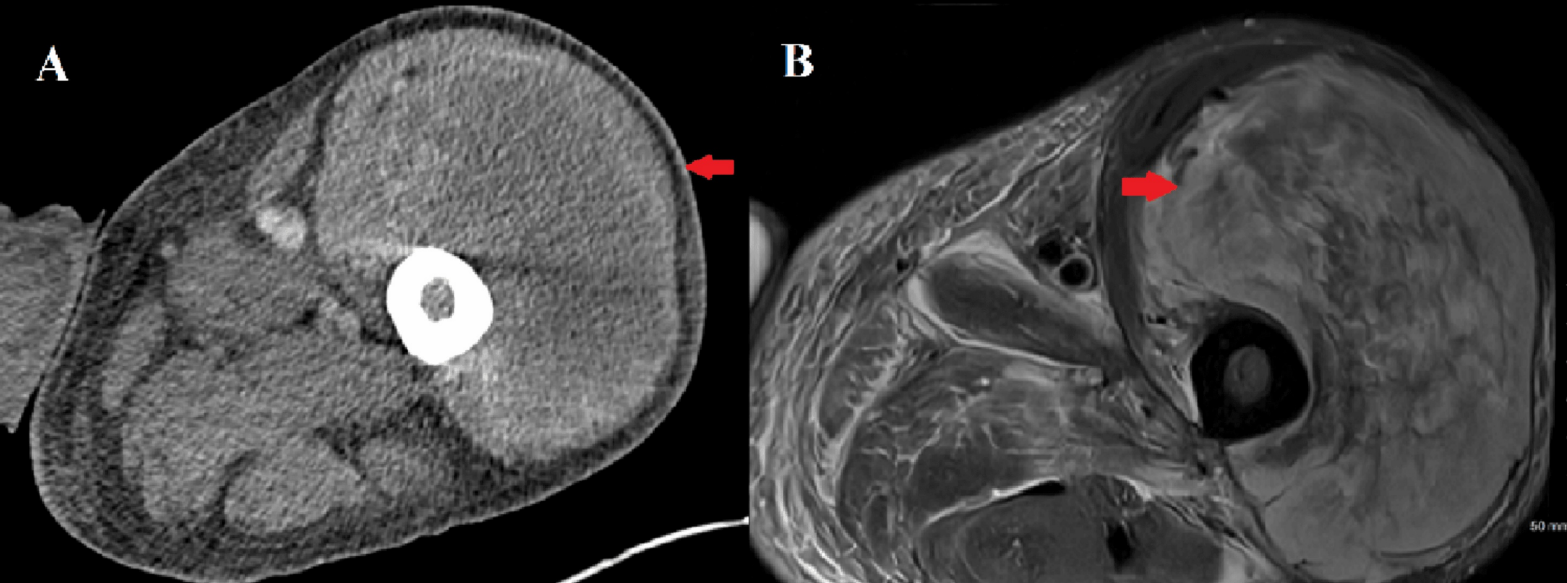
The CT scan and MRI of the patient A: CT scan in axial view reveals a left thigh mass in the anterior compartment measuring 11 x 16 x 11 cm (red arrow); B: MRI in axial view reveals a heterogenous peripherally enhancing mass in the anterior compartment of the thigh, predominantly in the vastus lateralis musculature, measuring 15.2 cm (red arrow)
